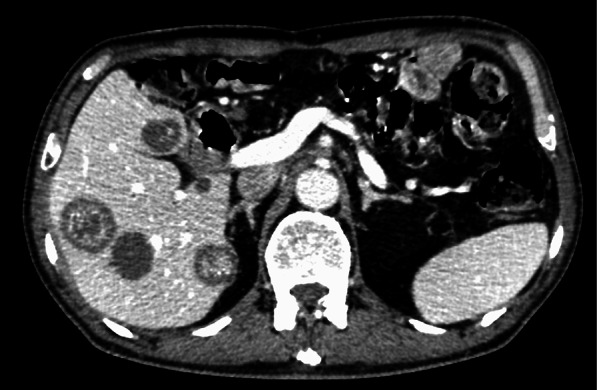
Contrast-enhanced CT. Multiple low-density areas in the liver were revealed. Cystoid nodes and irregular tumors with internal high density were found
